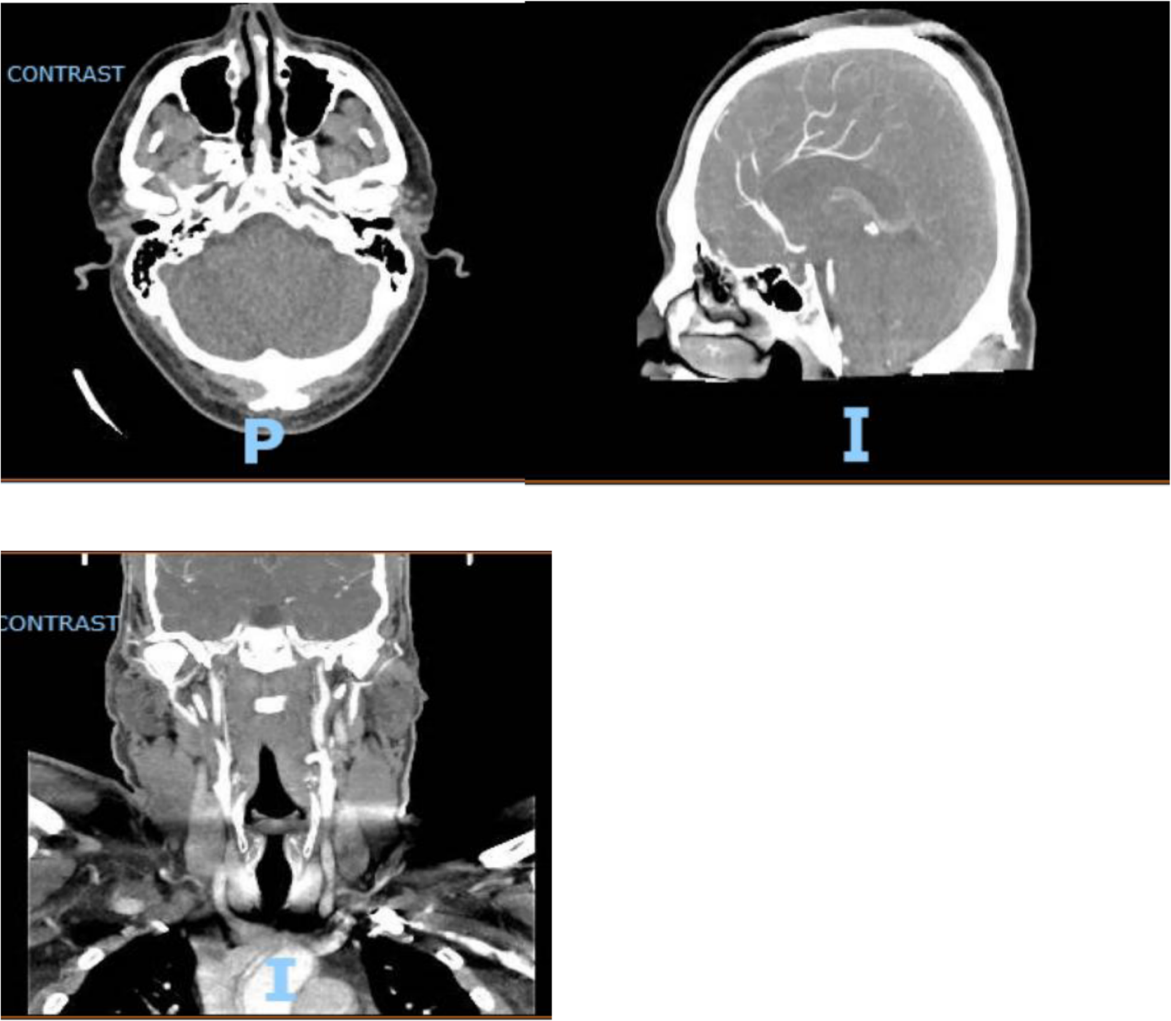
CT angiogram with IV contrast head and neck with patent circulation. A Axial (transverse) plane of head. B Sagittal plane of head. C Coronal plane of head
